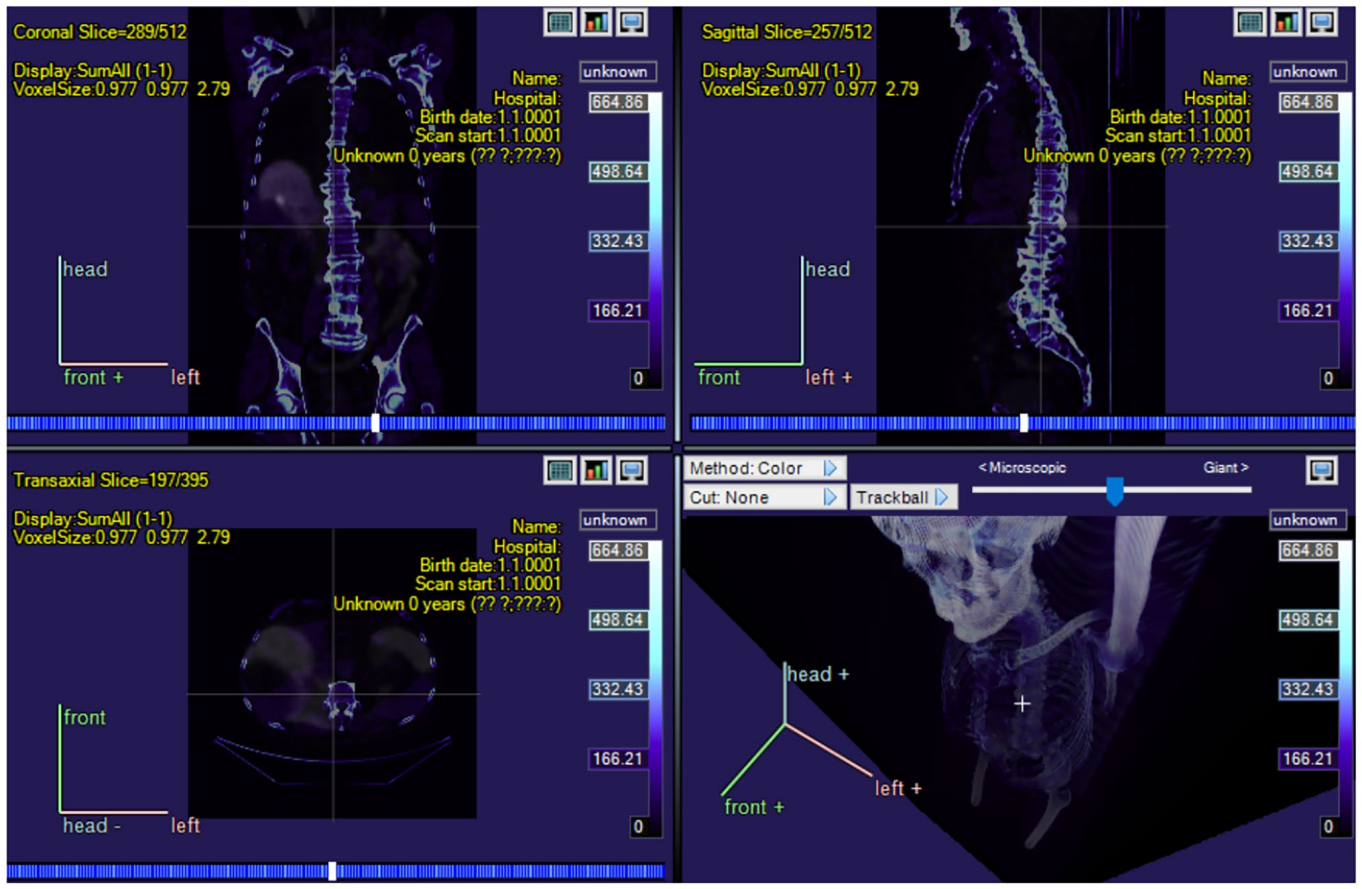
A screenshot of a whole-body PET/CT image of a prostate cancer patient opened in Carimas with color function called Ice, showing the coronal, the sagittal, and the transaxial directions and image modality fusion
